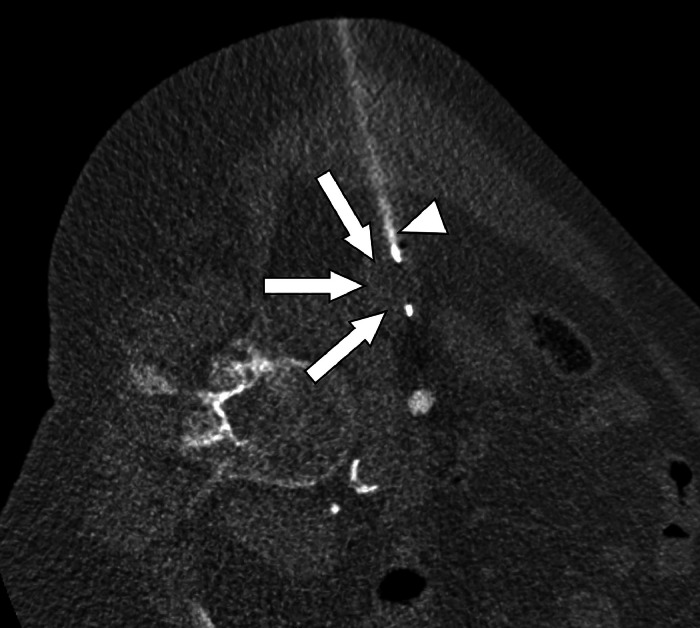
CT-guided biopsy with biopsy needle (white arrowhead) placed in the suspected mass on the lower pole of the right kidney (white arrows), on March 16, 2023. Unenhanced CT in left lateral decubitus position.
